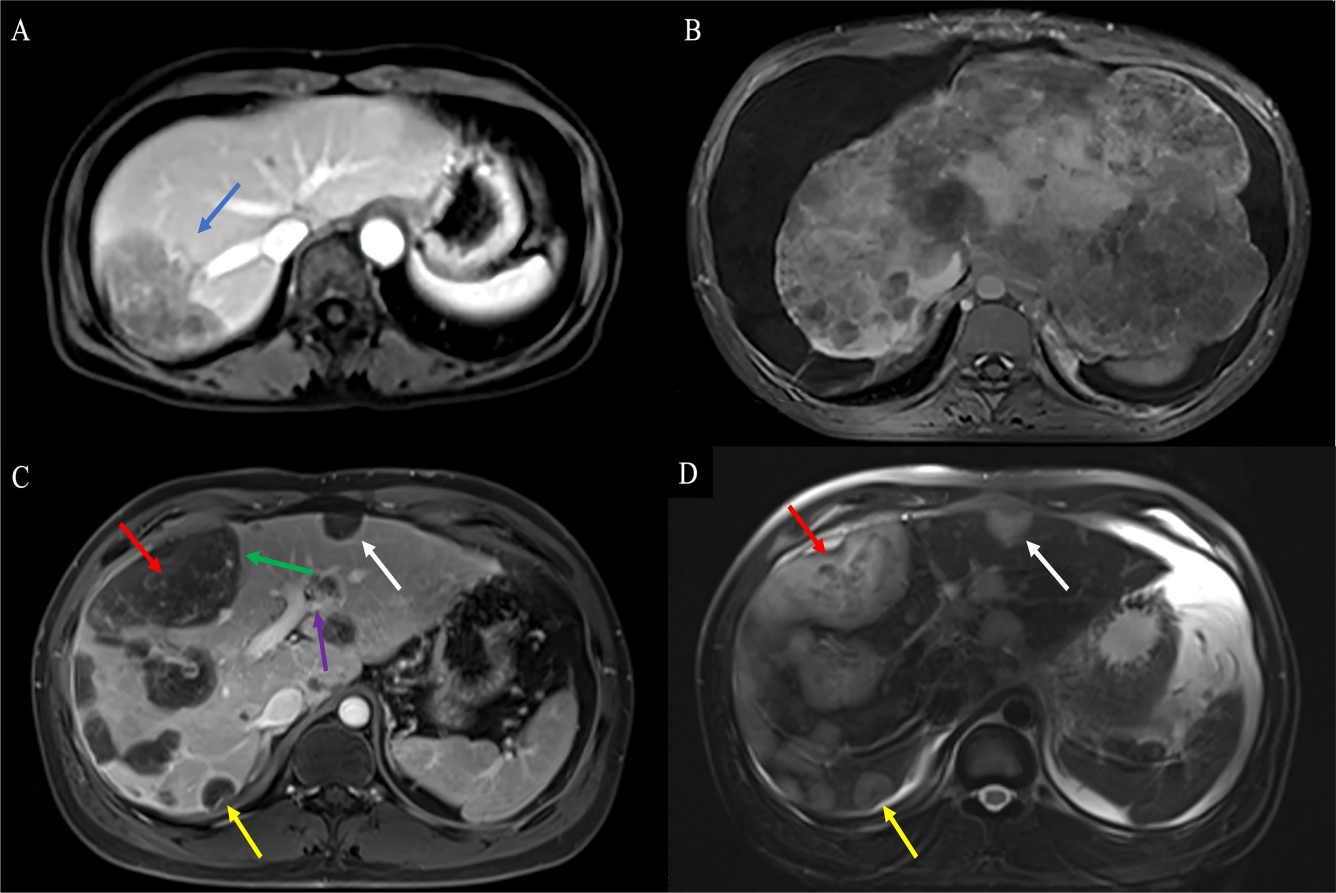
Findings of HEHE in contrast-enhanced MRI. (A) T1WI of contrast-enhanced MRI demonstrated a uninodular tumor with the characteristic “lollipop sign” (blue arrow). (B) T1WI of contrast-enhanced MRI demonstrated extremely a heterogeneous enhancement diffuse hypo-signal of varying sizes with hepatomegaly, RPV, and RHIVC compression. (C) Axial T1WI demonstrated predominantly peripherally located central hypointensity (red arrow) with peripheral high-signal rim (green arrow) and characteristic hepatic capsular retraction (yellow arrow). The LPV was infiltrated (purple arrow). Smaller lesions demonstrated uniform hypointensity with clear margins (white arrow), while larger lesions showed heterogeneous enhancement hypointensity (red arrow) (D). Axial fat-suppressed T2WI demonstrated hyperintensity in tumor. Smaller lesions showed uniform hyperintensity (white arrow), while larger lesions showed heterogeneous enhancement hyperintensity (red arrow). HEHE, hepatic epithelioid hemangioendothelioma; MRI, magnetic resonance imaging; T1WI, T1-weighted image; RPV, right branch portal vein; RHIVC, retro hepatic inferior vena cava; T2WI, T2-weighted image.
